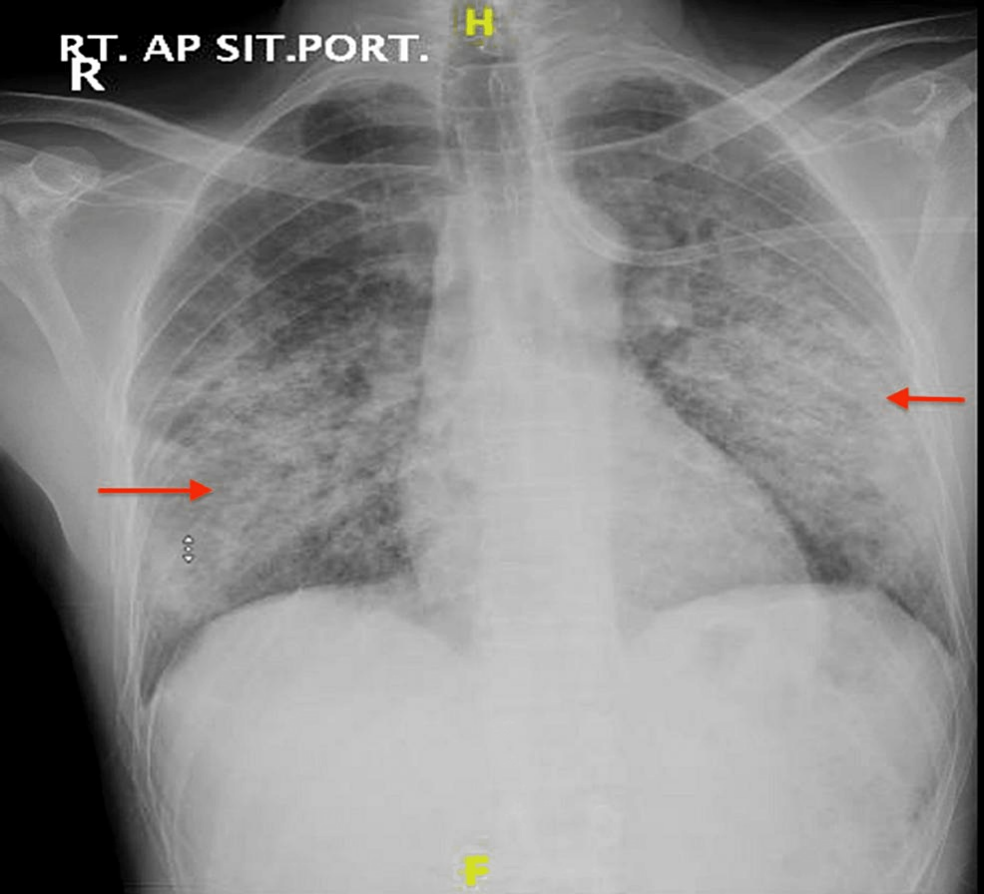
Chest X-ray obtained in the ICU
